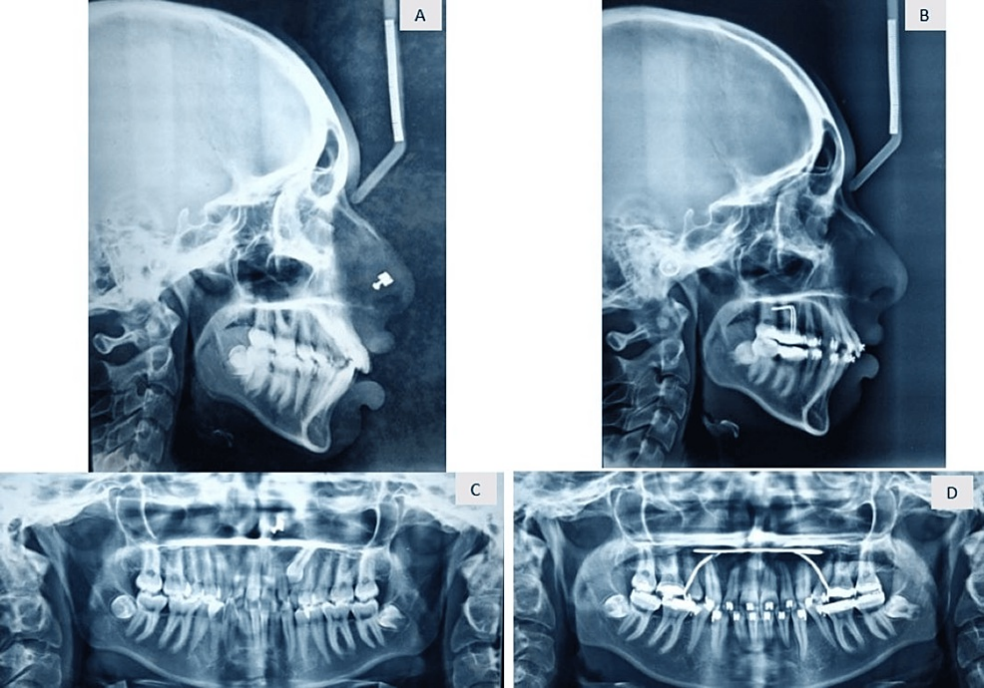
Comparison of pre- and post-treatment radiographs: pre-treatment: (A) OPG and (C) lateral cepahlogram and post-treatment: (B) OPG and (D) lateral cepahlogram OPG: Orthopantomogram
